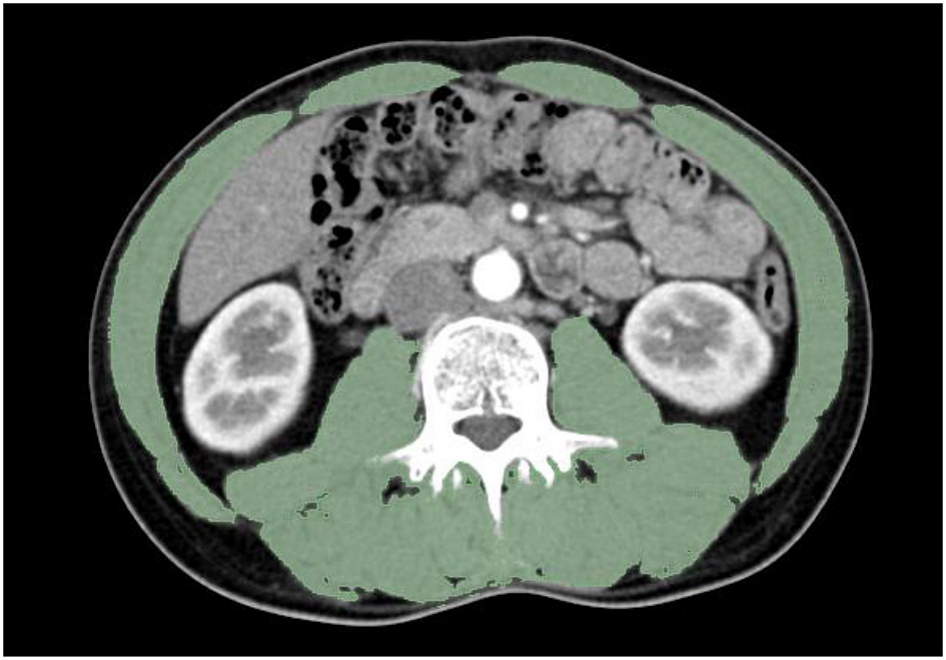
Example of a computed tomography image.
